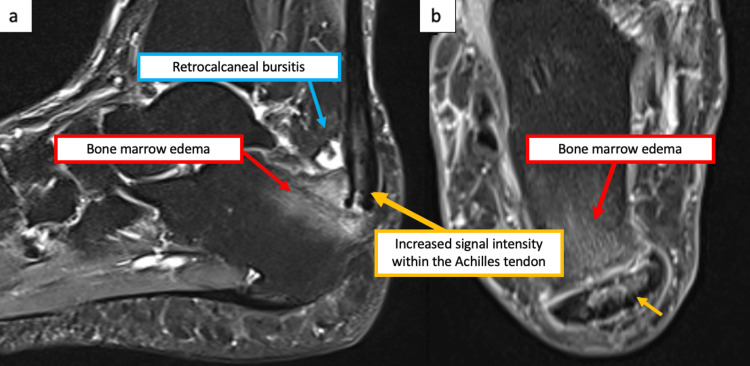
MRI features of calcaneo-Achilles impingement in Haglund syndrome Sagittal (a) and axial (b) proton density fat-suppressed MRI sequences demonstrating hyperintense signal within the posterosuperior calcaneus (red arrow), compatible with bone marrow edema; increased signal intensity at the Achilles tendon insertion (yellow arrow) suggestive of insertional tendinopathy; and minimal fluid signal within the retrocalcaneal bursa (blue arrow). MRI: magnetic resonance imaging
